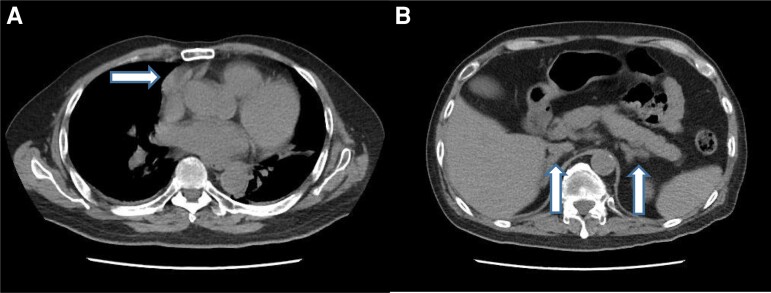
Computed tomography at presentation. A, ACTH-secreting right anterior mediastinal mass (arrow). B, bilateral adrenal hyperplasia (arrows).
